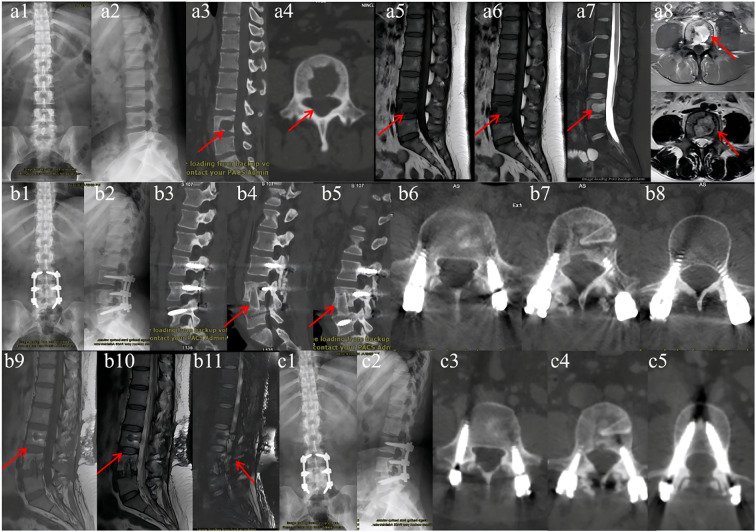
Preoperative and postoperative follow-up imaging of a patient with *coagulase-negative Staphylococcus* infection treated with direct pedicle screw fixation involving the infected vertebrae. Preoperative imaging: (a1, a2) Anteroposterior and lateral radiographs. (a3, a4) Sagittal CT images revealing bone destruction and a large cavity in the L4 vertebral body (arrows). (a5–a8) Sagittal and axial MRI demonstrating abscess formation in the L4 vertebral body and adjacent intervertebral space, with satisfactory vertebral body height (arrows). Postoperative imaging at 9 months: (b1, b2) Anteroposterior and lateral radiographs. (b3–b8) Sagittal and axial CT images showing good bone graft fusion at the site of previous bone destruction and cavity, with internal fixation in good position (arrows). (b9–b11) Sagittal MRI demonstrating no abscess recurrence (arrows). Postoperative imaging at 12 months: (c1, c2) Anteroposterior and lateral radiographs. (c3–c5) Sagittal CT images revealing good position of internal fixation and satisfactory bone graft fusion.
